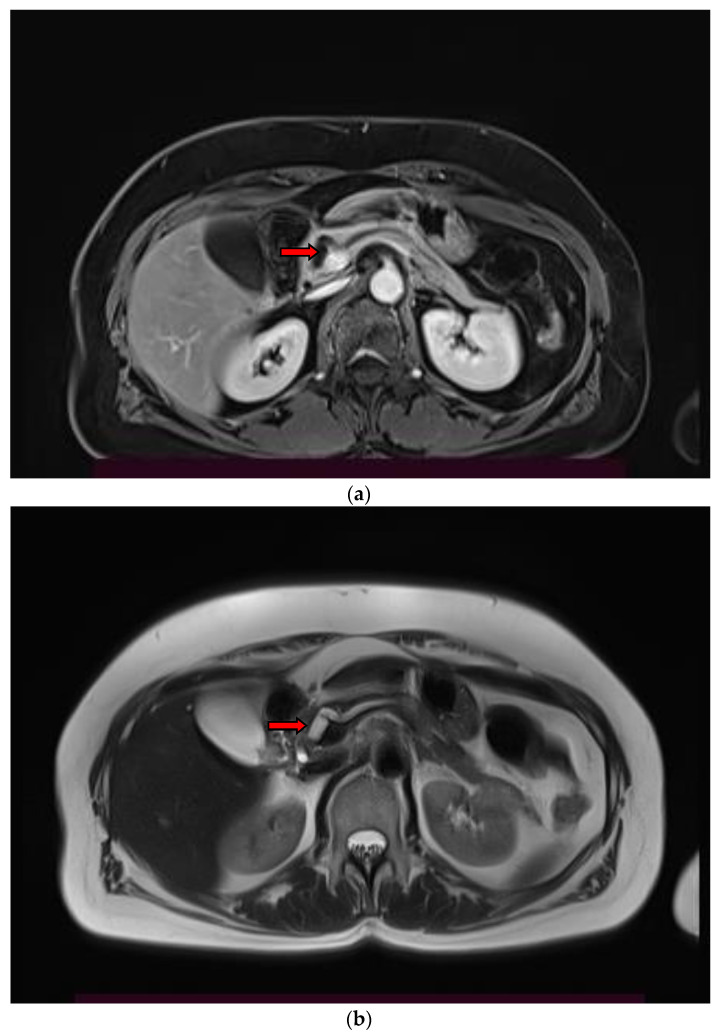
Contrast-enhanced T1-w MR image (a) and T2-w MR image (b), demonstrate a marked dilatation of the main pancreatic duct in the head and neck of the pancreas, consistent with a segmental main duct IPMN (arrow).
